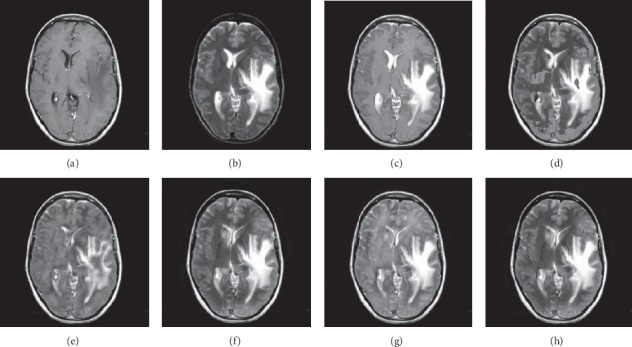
CT and MR medical image fusion results. (a) CT original image. (b) MR original image. (c) NSST-PAPCNN. (d) CSR. (e) MST-SR. (f) NSCT-SR-PCNN. (g) SR-PCNN. (h) Proposed.
